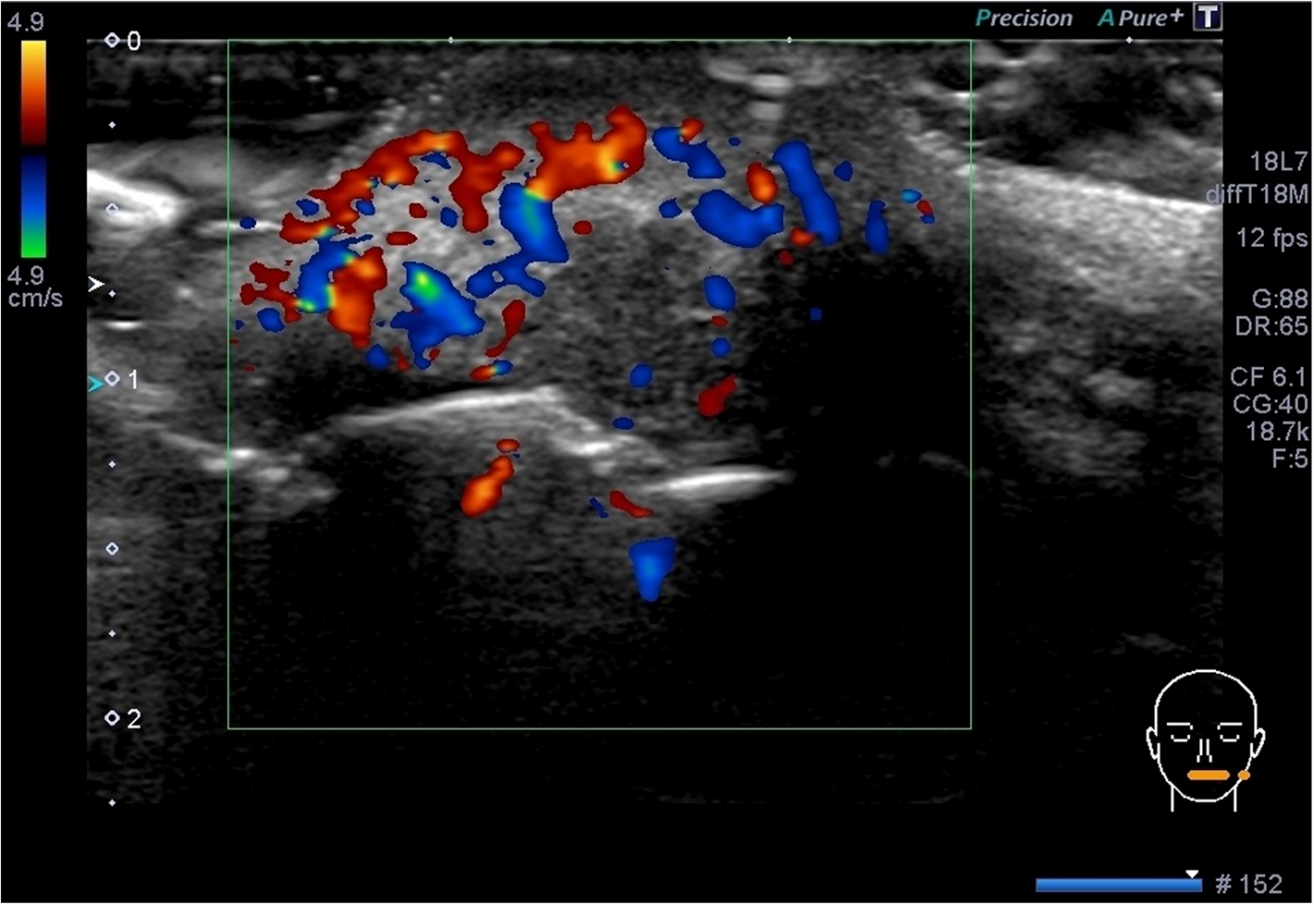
A color Doppler sonogram of the tumor-like lesion. A color Doppler sonogram of the tumor-like lesion revealed very abundant blood flow in the heterogeneous sites and poor blood flow in the hypoechoic part of the tumor-like lesion
